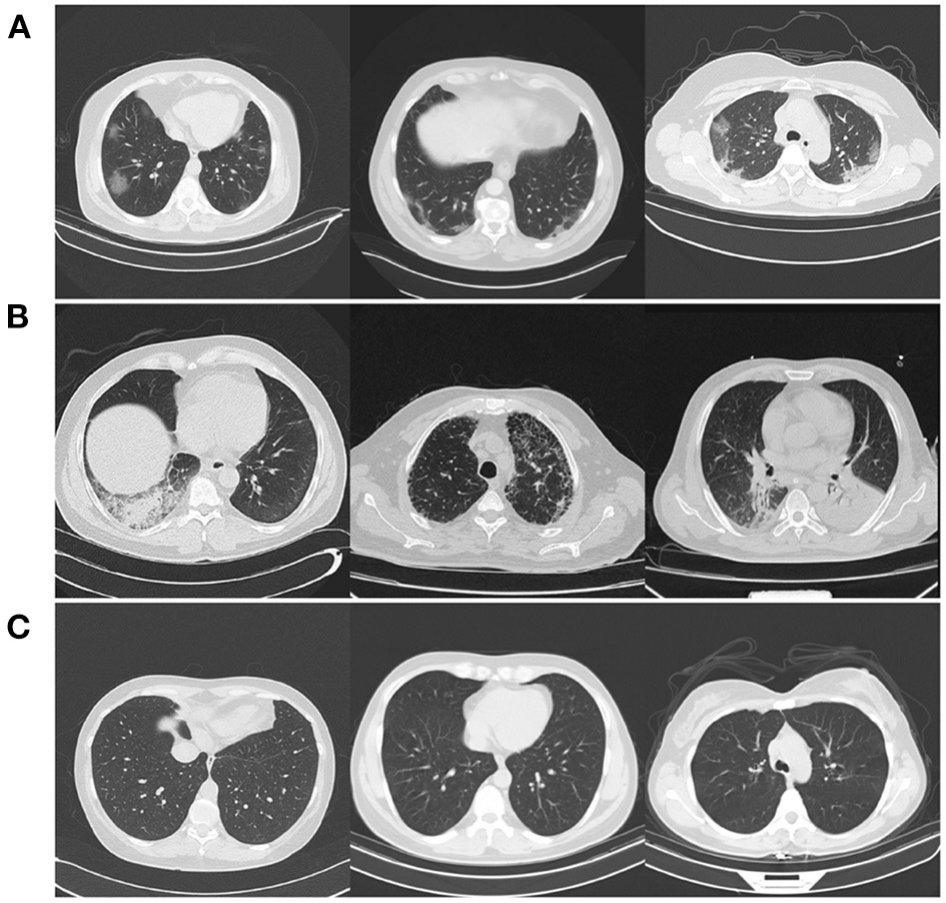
Example CT images from the COVIDx CT-2 benchmark datasets from each type of infection: (A) novel coronavirus pneumonia due to SARS-CoV-2 infection (NCP), (B) common pneumonia (CP), and (C) normal controls.
